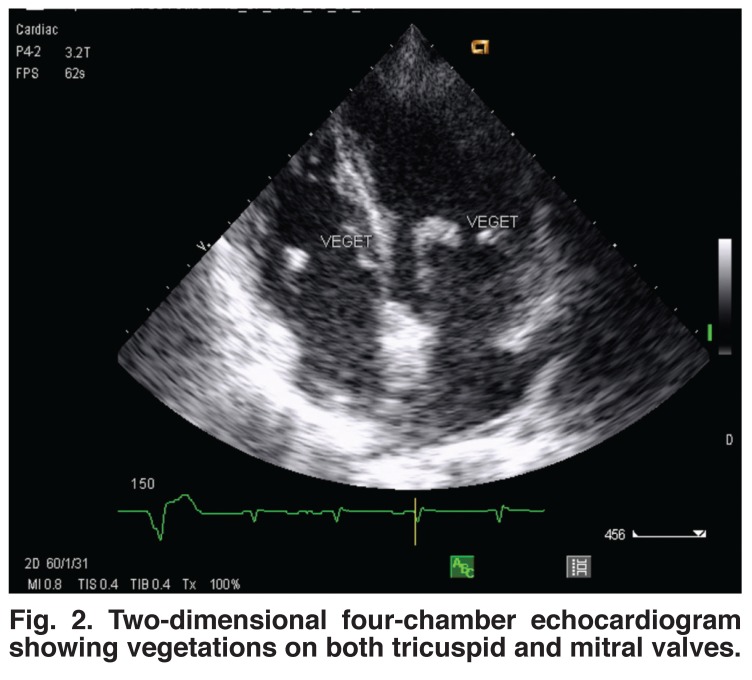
Two-dimensional four-chamber echocardiogram showing vegetations on both tricuspid and mitral valves.
